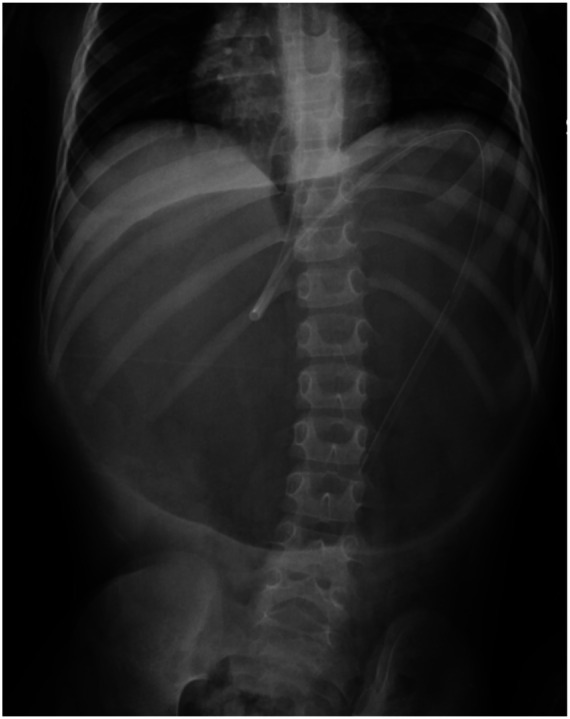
Plan abdominal x-ray showed massive gastric dilatation, NG tube in place.
